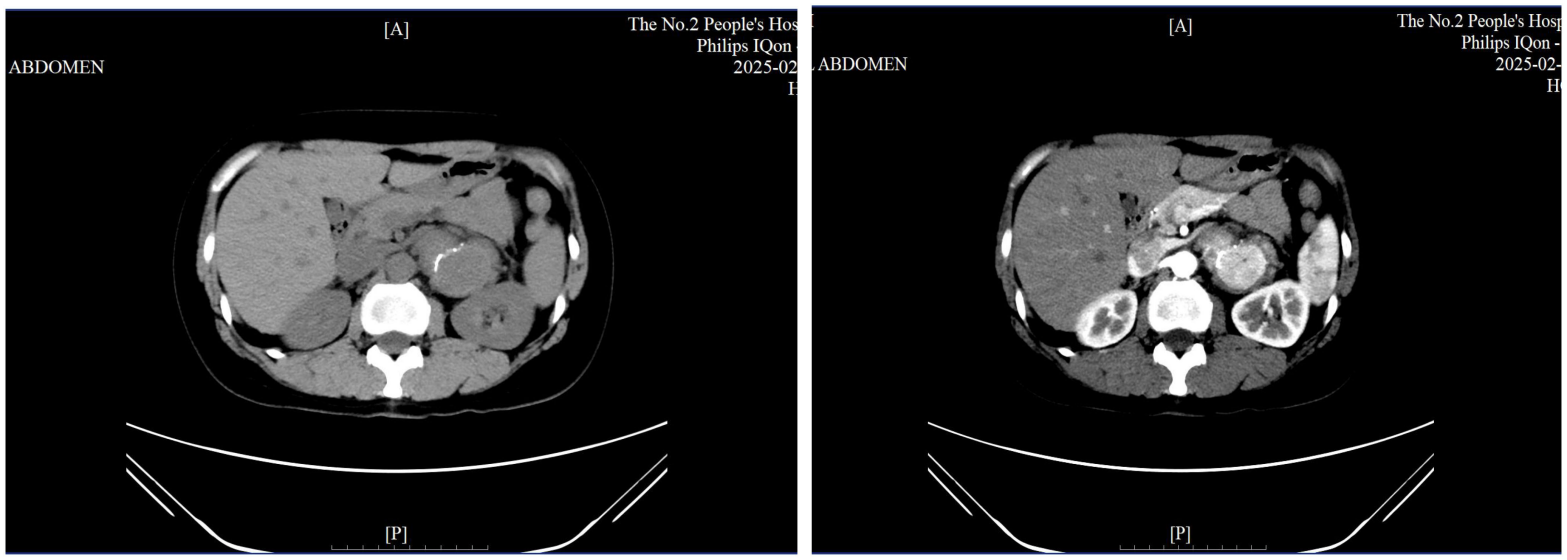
Contrast-enhanced CT scan showing a well-defined 5.3 cm lesion in the left adrenal region with calcification.
